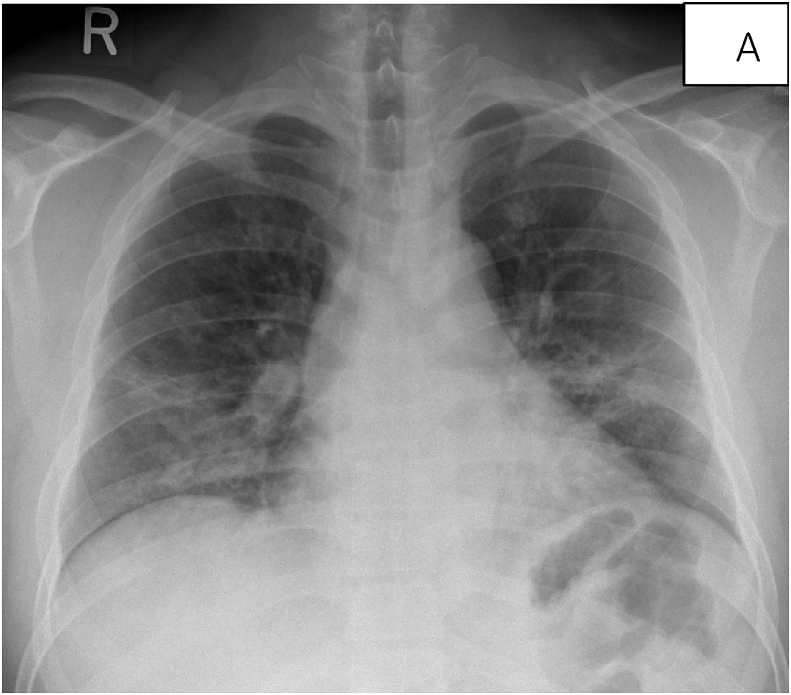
Chest radiograph: Bilateral lower and mid zone infiltrates.
